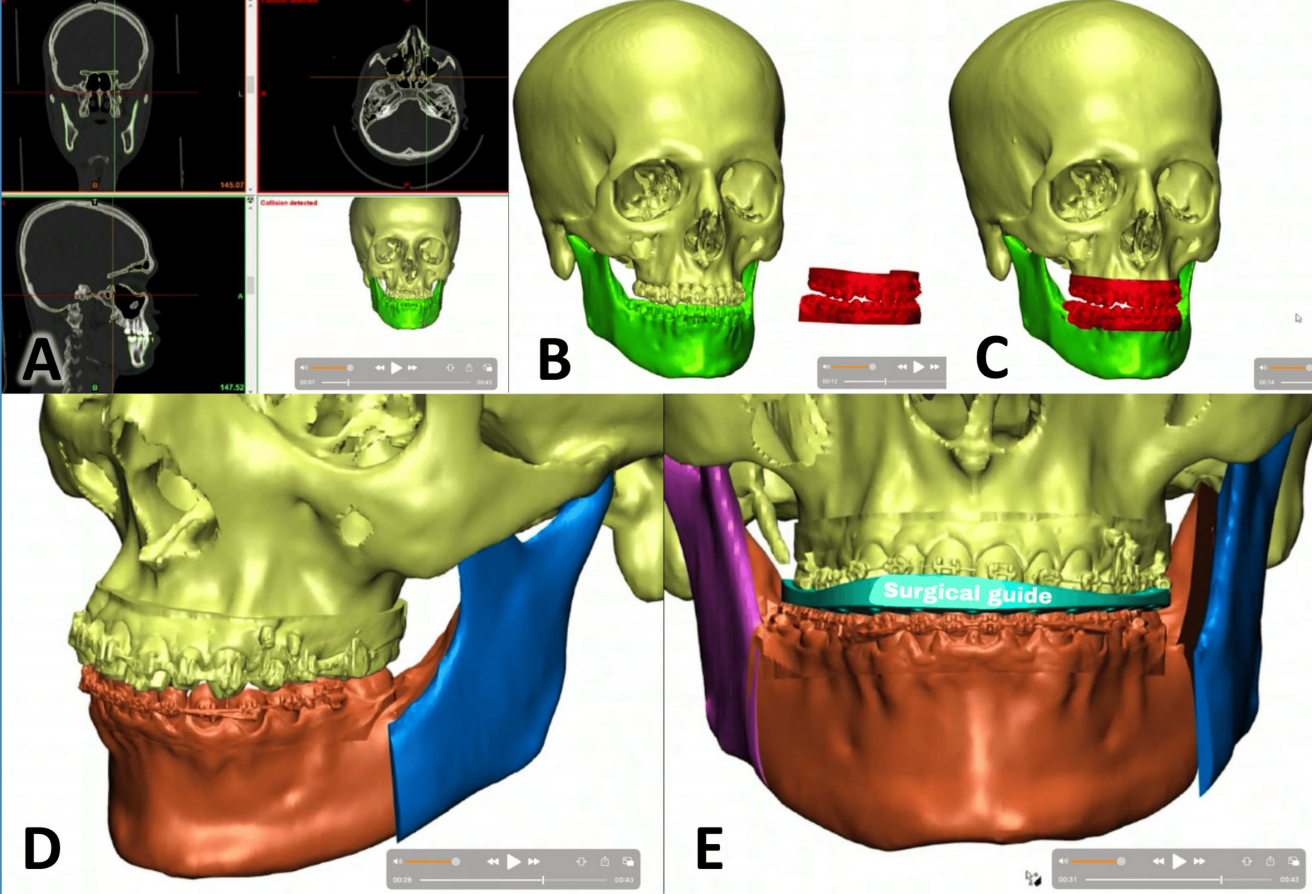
The stages of the virtual surgical planning (VSP) in orthognathic surgery using 3D patient records. A) A cone-beam computed tomography (CBCT) scan of the face is performed, including axial, sagittal, coronal, and 3D rendered views; B) the scanning of the teeth with an intraoral 3D camera before transferring the data to the 3D model of the skull; C) the 3D scan is transferred to the radiographic model, involving a matching procedure where the teeth and parts of the bone on the model are replaced with the precise jaw relationships obtained from the 3D scan; D) the virtual 3D planning of the surgical interventions according to the planned movements of one or both jaws; E) a surgical guide is fabricated to accurately transfer the newly established jaw relationship for implementation during surgery.
